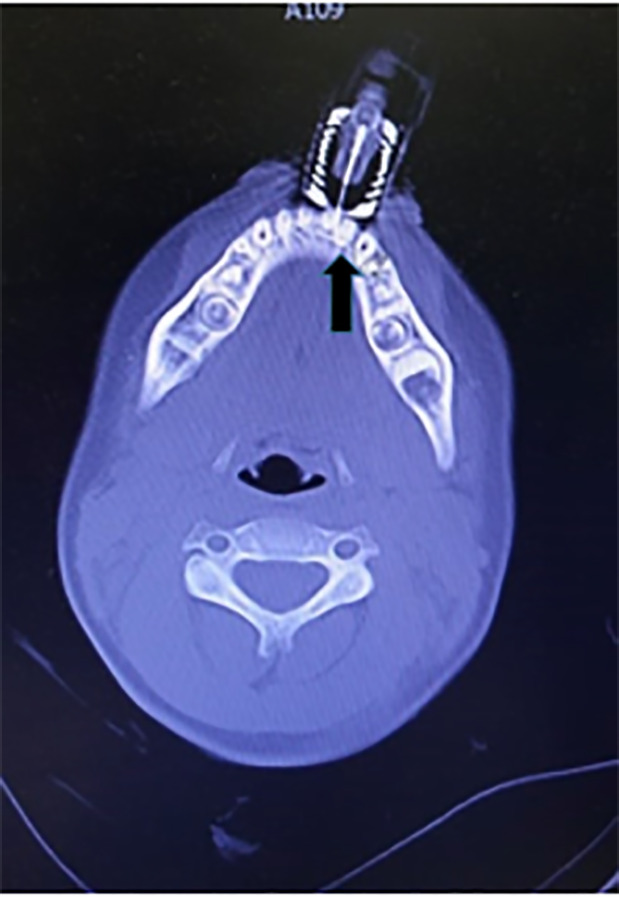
Maxillofacial computed tomography with axial view reconstruction
